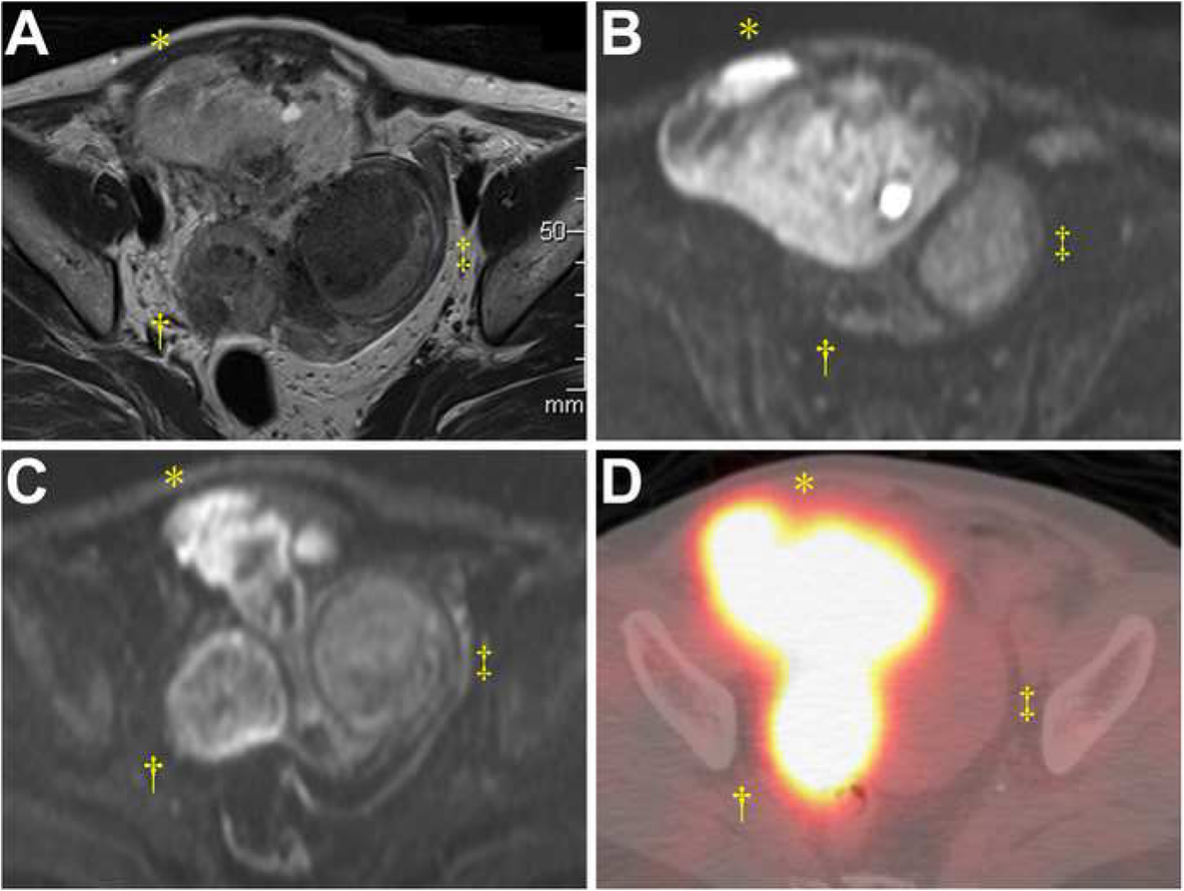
Imaging of the uterine tumor composed of three components. a Pelvic axial T2-weighted magnetic resonance imaging disclosing a mass with heterogenous, mixed low and high intensity (*) and another mass with mostly low intensity (†) attached to the right-anterior and right-posterior serosa, respectively, and the remaining low-intensity intramural mass in the fundus (‡). b and c Diffusion-weighted images showing high intensity in the right-anterior tumor (*) and slightly high intensity in the right-posterior (†) and the fundal (‡) tumors (d) Positron emission tomography/computed tomography fusion image demonstrating high ^18^F-fluorodeoxyglucose uptake of the right-anterior and right-posterior tumors (*, †) in contrast to no uptake of the fundal tumor (‡)
